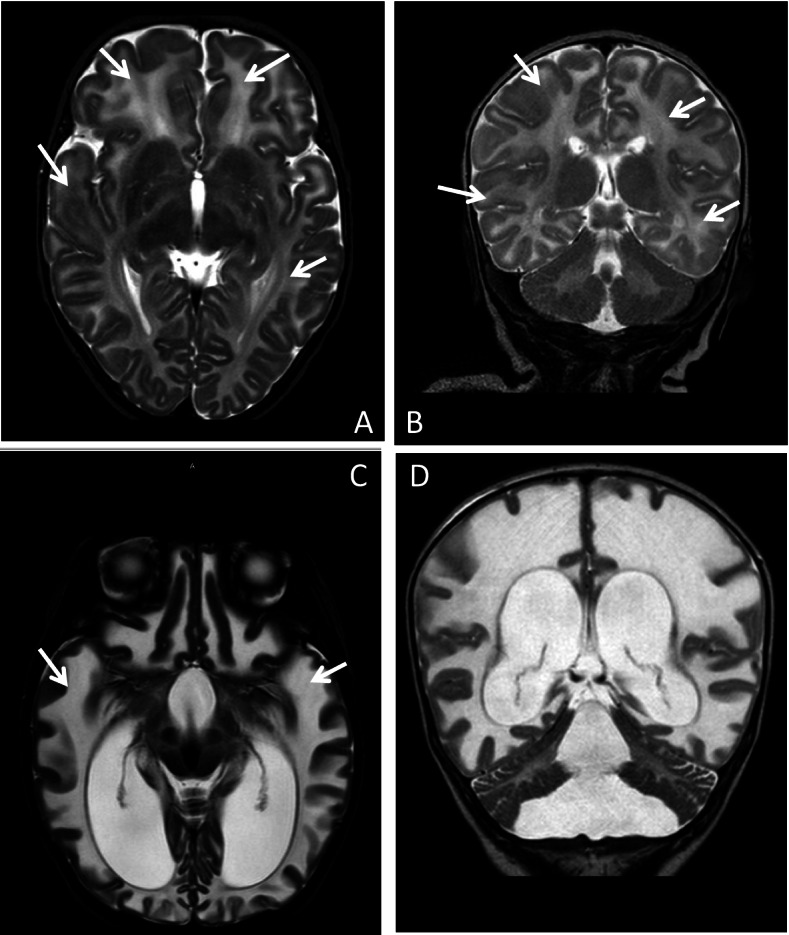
A and B showed T2 sequences -MRI at three months of life; C and D were the T2 sequences- MRI performed at ten months of life: in A and B white matter appears extensively hyperintense with absence of regular myelination. At ten months of life (C and D) T2-MRI shows increased hyperintensity of both cortical and deep white matter with significant dilatation of the ventricular system
